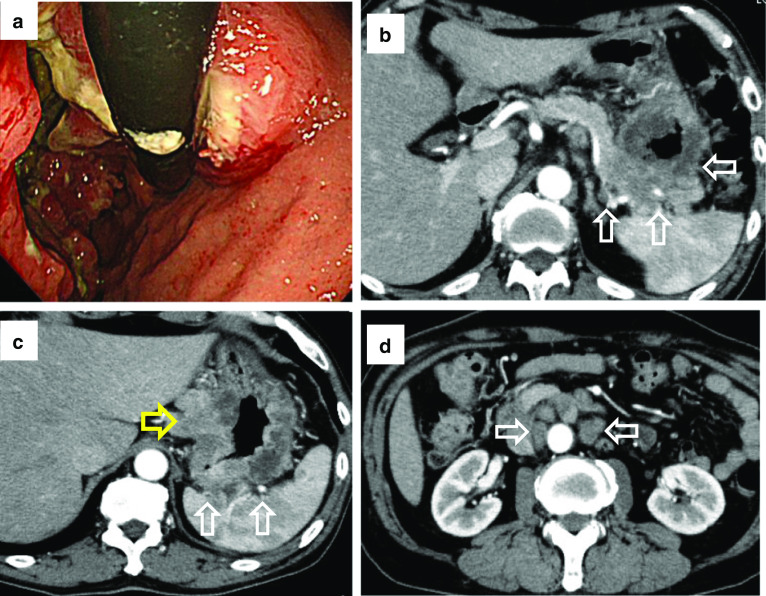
Endoscopic and computed tomography (CT) images before nivolumab therapy. a First gastric endoscopy examination showing Borrmann type 3 gastric cancer occupying the entire circumference of the gastric body. b First CT image showing the primary tumor invading the pancreatic tail (white arrows). c The spleen was also invaded (white arrows), and the lesser curvature lymph nodes were enlarged (yellow arrows). d Enlarged para-aortic lymph nodes (white arrows)
